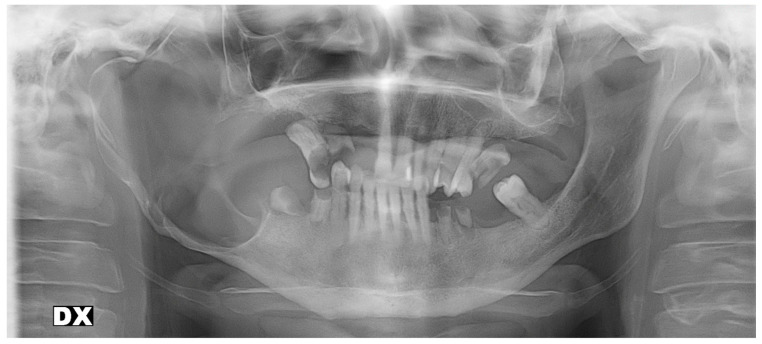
Pre-surgery panoramic X-ray showing a wide radiolucent lesion extending from dental elements 4.5 to the entire right mandible ramus involving the right mandibular body.
